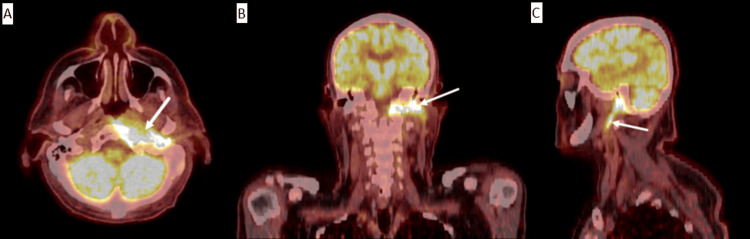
Positron emission tomography (PET) scan showing a fluorodeoxyglucose (FDG)-avid lesion (white arrow) involving posterior left nasopharynx, skull base, and tracking along the osseous course of the facial nerve with associated osseous erosion in transverse (A), coronal (B), and sagittal (C) views.
